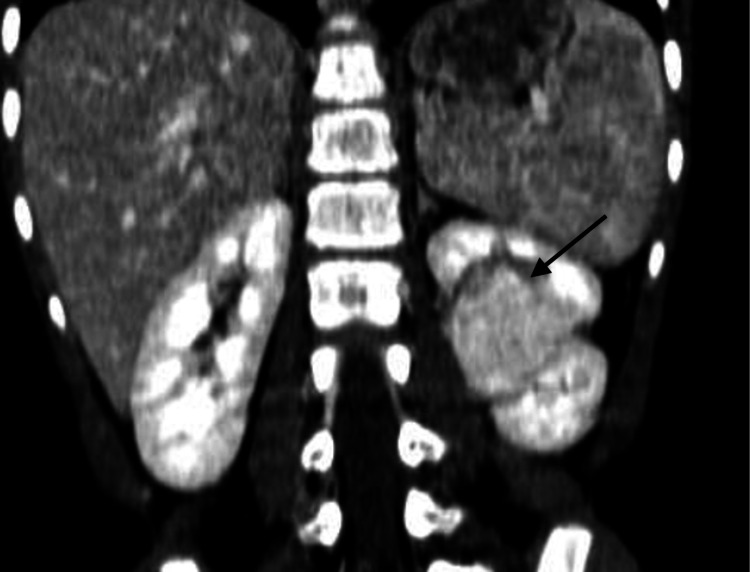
Coronal CT image in the nephrographic phase showing homogeneous enhancement of the lesion
